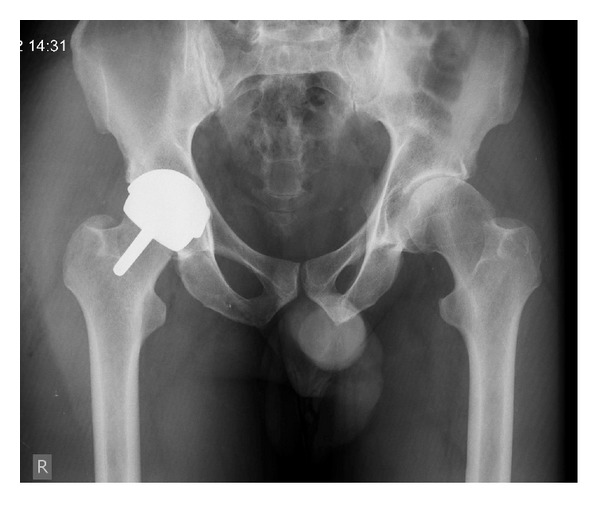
Postoperative radiograph after right Birmingham Hip Resurfacing. Left hip demonstrates features consistent with cam type femoroacetabular impingement and secondary osteoarthritis.
